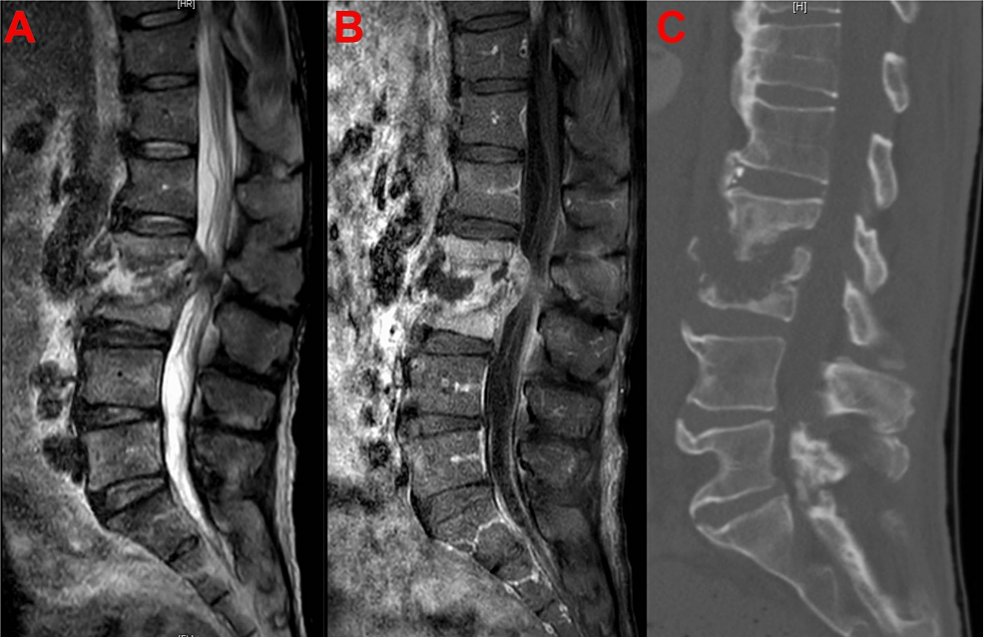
(A) Midsagittal T2-weighted MRI without contrast. (B) Midsagittal T1-weighted MRI with contrast. (C) Midsagittal CT scan without contrast. Imaging prior to initial intervention showing L2 and L3 discitis-osteomyelitis with intradiscal abscess causing cord compression.
